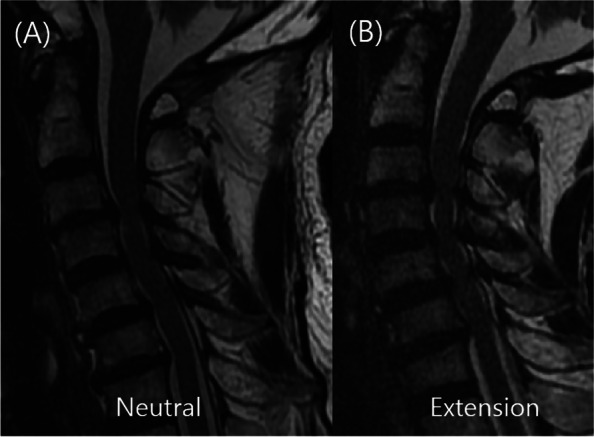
Case 41. Disagreement regarding the segments that needed a surgical treatment for CSM. A Neutral sagittal MR imaging of the cervical spine. B Extension sagittal MR of the cervical spine. From the neutral image (A), all reviewers suggested that the C3–4 segment is affected with CSM, and that it is where surgical treatment is indicated. However, from the extension image (B) they suggested that the C3–7 segments is rather involved and needs a surgical treatment
